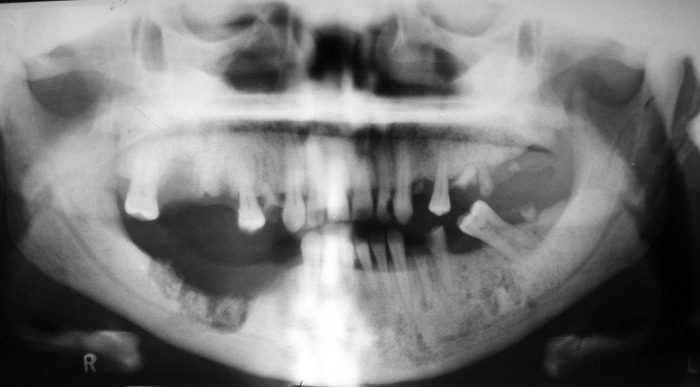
Unfortunately, a delay of three months to refer the patient for surgical treatment resulted in severe osteomyelitis in the region and spread of the infection into the submandibular space (Figure 2). Admission hematologic tests included red blood cell count, white blood cell count, platelet count, hemoglobin, and hematocrit; red blood cell indices were within the normal limits, as were erythrocyte sedimentation rate and coagulation profile. Posteroanterior (PA) chest x-ray revealed a uniform increase in bone density throughout the thoracic cage and both clavicular bones, with no abnormal finding in the chest parenchyma on either side (Figure 3).
